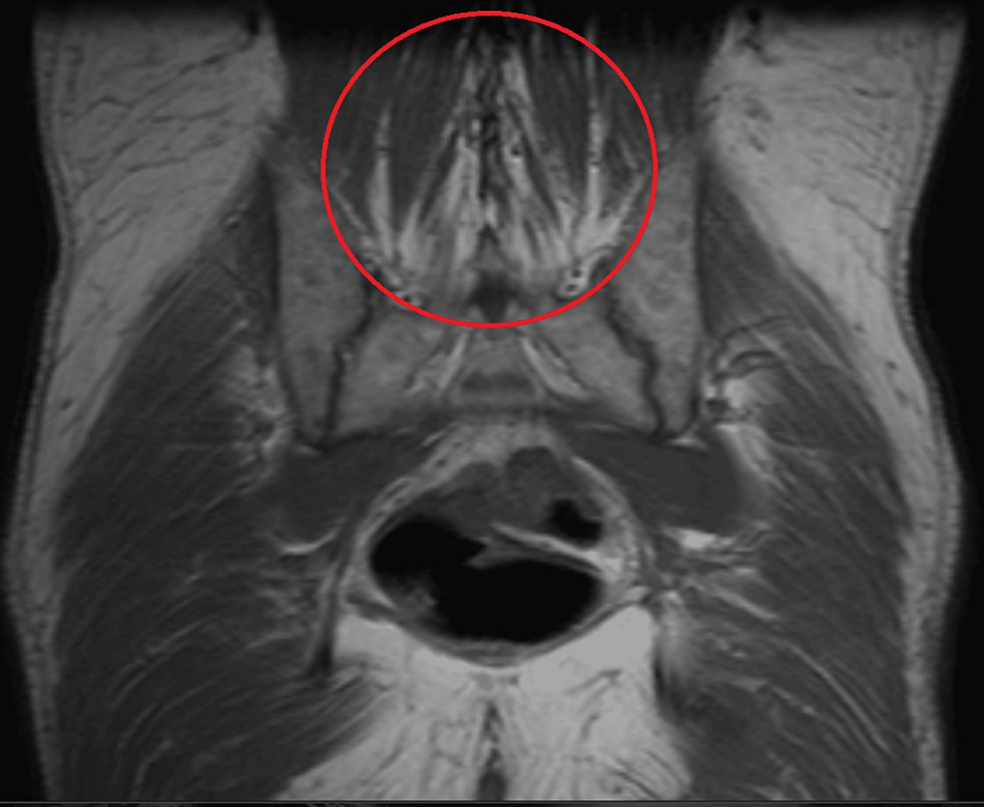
MRI of pelvis without contrast. The red circle demonstrates an increased signal in the paraspinal muscles and bilateral abductor muscles without any evidence of masses or fluid collections.
